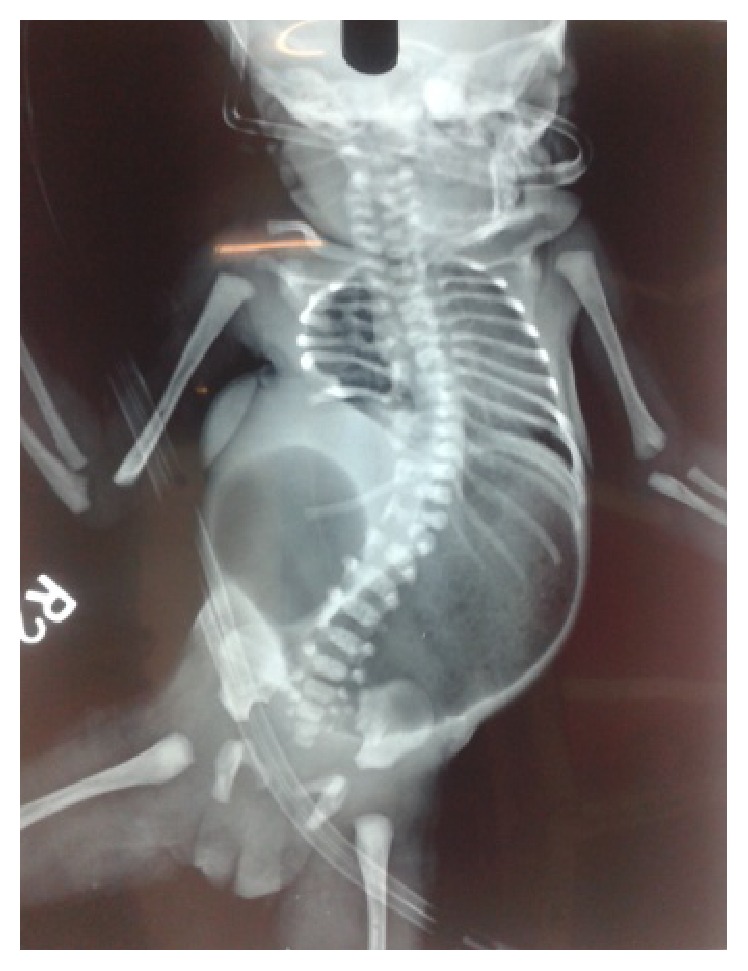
X-ray showing congenital lumbar hernia associated with duodenal atresia and lumbocostovertebral syndrome.
